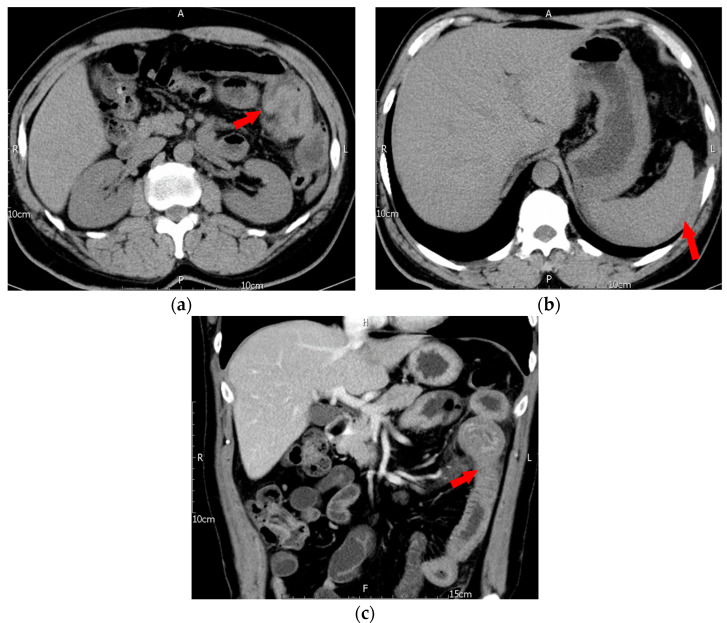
First episode of intramural hematoma of jejunum. (a) Axial non-contrast CT showed marked segmental wall thickening of the proximal jejunum with diffuse hyperdensity (arrow), (b) axial non-contrast CT showed perisplenic hemoperitoneum (arrow), (c) coronal reformatted contrast-enhanced CT showed contrast enhancement of the inner mucosa, but there was no contrast enhancement of the thickened wall of the corresponding proximal jejunum, which indicated the presence of an intramural hematoma (arrow).
